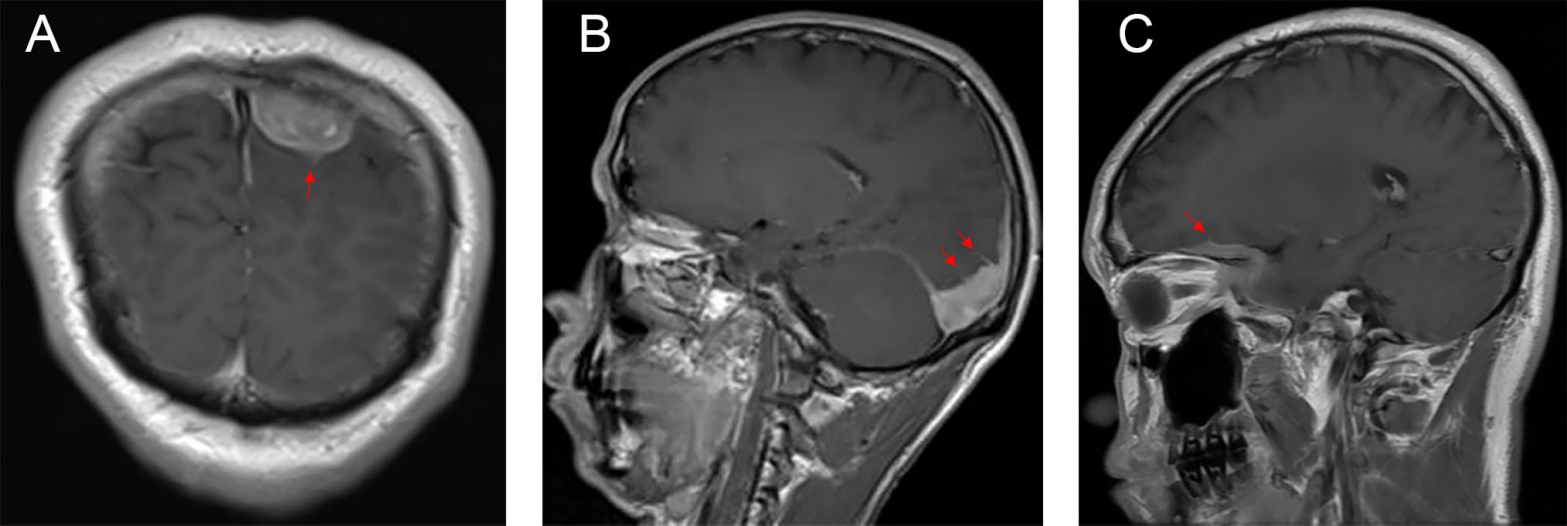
Postcontrast MRI images show that the irregularly thickened meningeal had a “pseudopodium” extending to the brain parenchyma (red arrow). (A) Coronal postcontrast T1WI. (B, C) Sagittal postcontrast T1WI.
